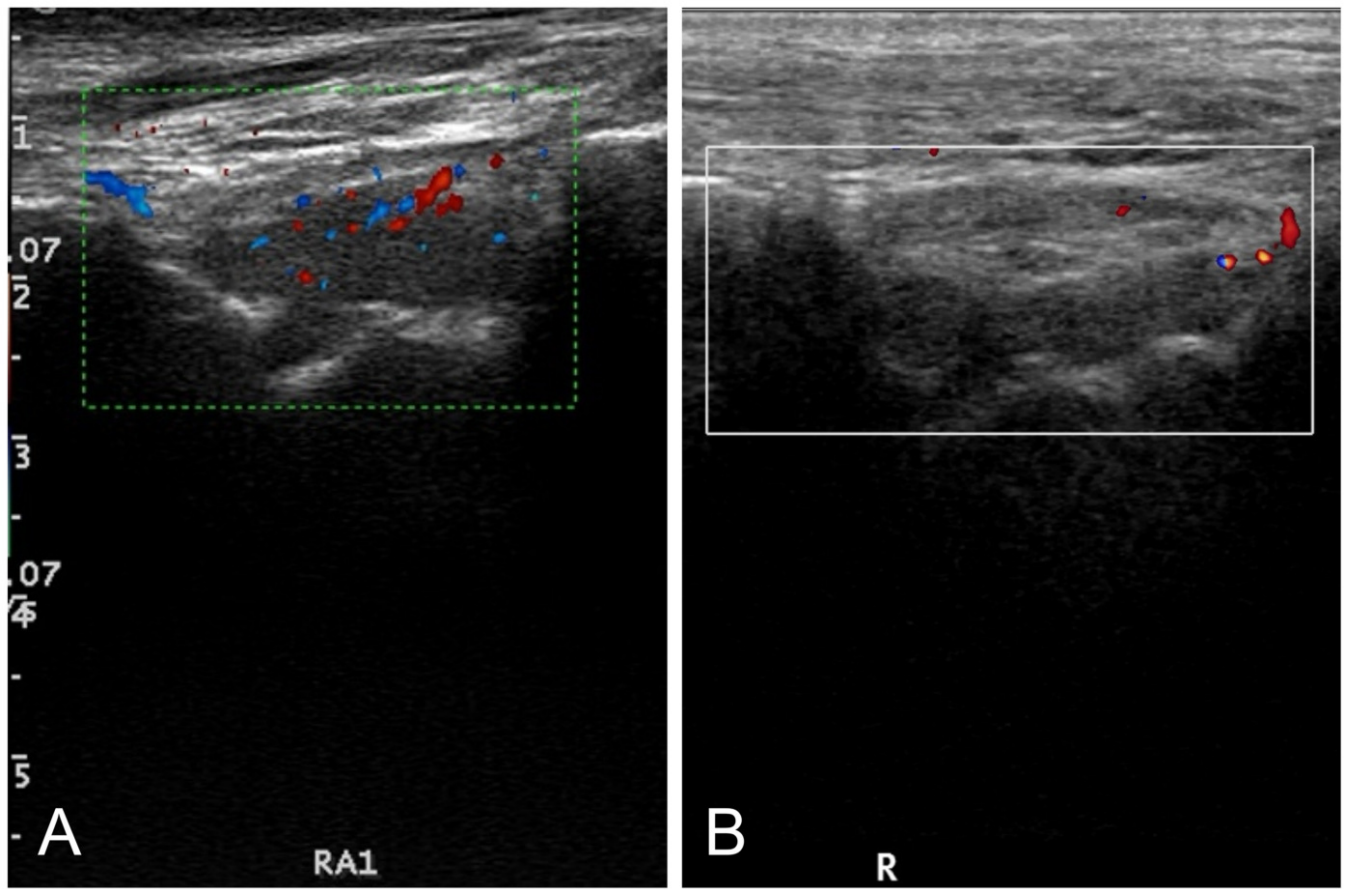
Ultrasonography of the right ankle joint before and after rehabilitation. (A) Before rehabilitation: synovial membrane thickening in the right ankle joint was observed, with the thickest part approximately 5.4 mm. Three-quarters of the joint cartilage was thinned, and the bone cortex was significantly irregular. CDFI: Linear blood flow signals were seen in the thickened synovial membrane. Right ankle joint synovial hyperplasia (2 points), synovial vascular proliferation (2 points), joint cartilage loss (3 points), bone destruction (2 points). (B) After rehabilitation: synovial membrane thickening in the right ankle joint was observed, with the thickest part approximately 5 mm. Three-quarters of the joint cartilage was thinned, and the bone cortex was significantly irregular. CDFI: Star-like blood flow signals were seen in the thickened synovial membrane. Right ankle joint synovial hyperplasia (2 points), synovial vascular proliferation (1 point), joint cartilage loss (3 points), bone destruction (2 points).
